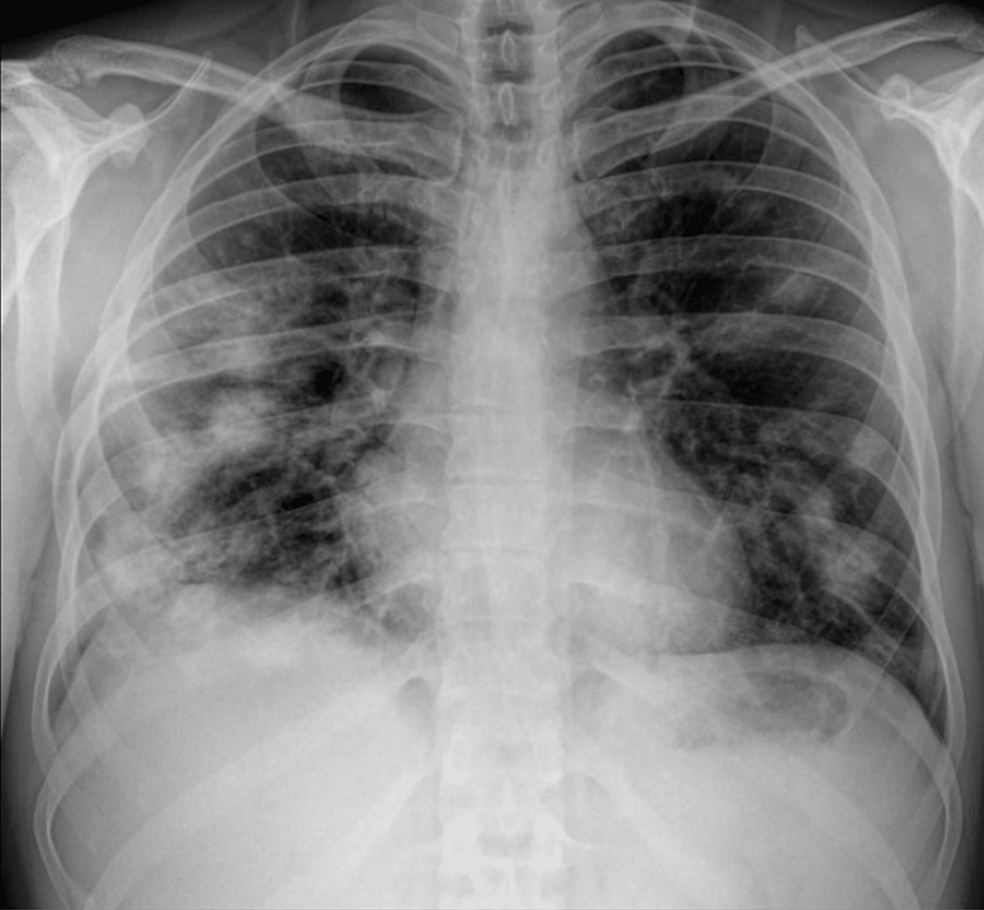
Chest X-ray (posterior-anterior view) upon admission to the ED.
